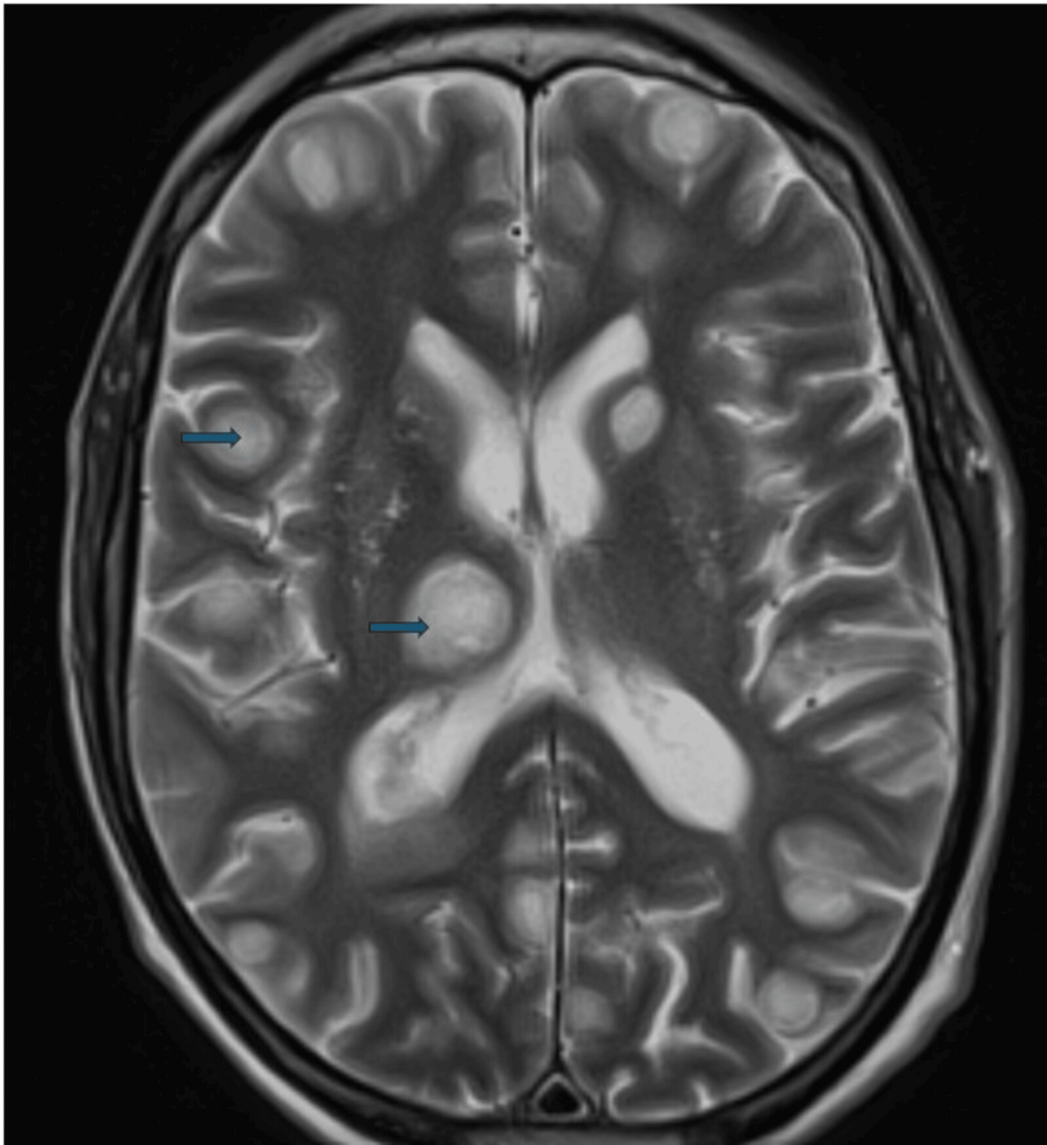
A transverse MRI of the head study section revealing multiple bilateral ring-enhancing lesions MRI: magnetic resonance imaging
